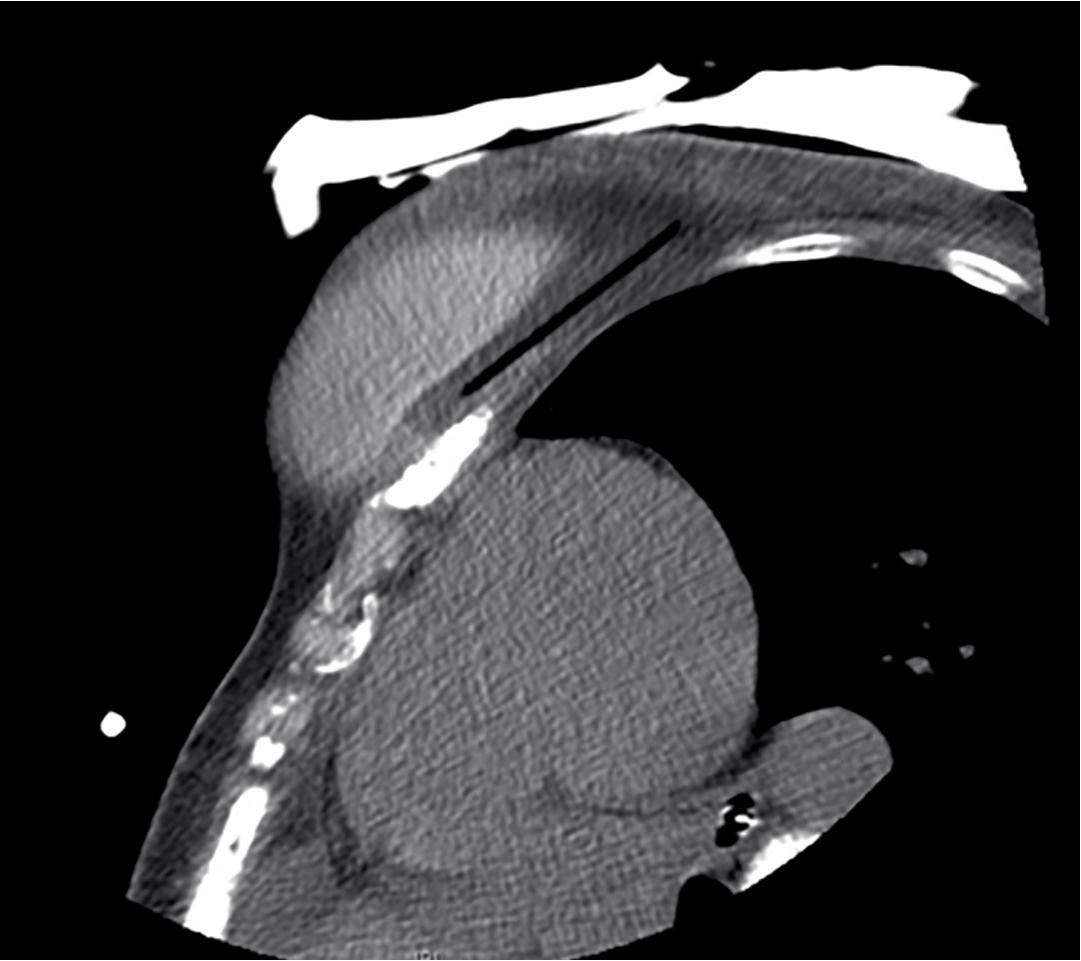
CT image during the second cryoablation demonstrates the cryoprobe's trajectory posterior to the implant. The ice ball extends into the implant.
